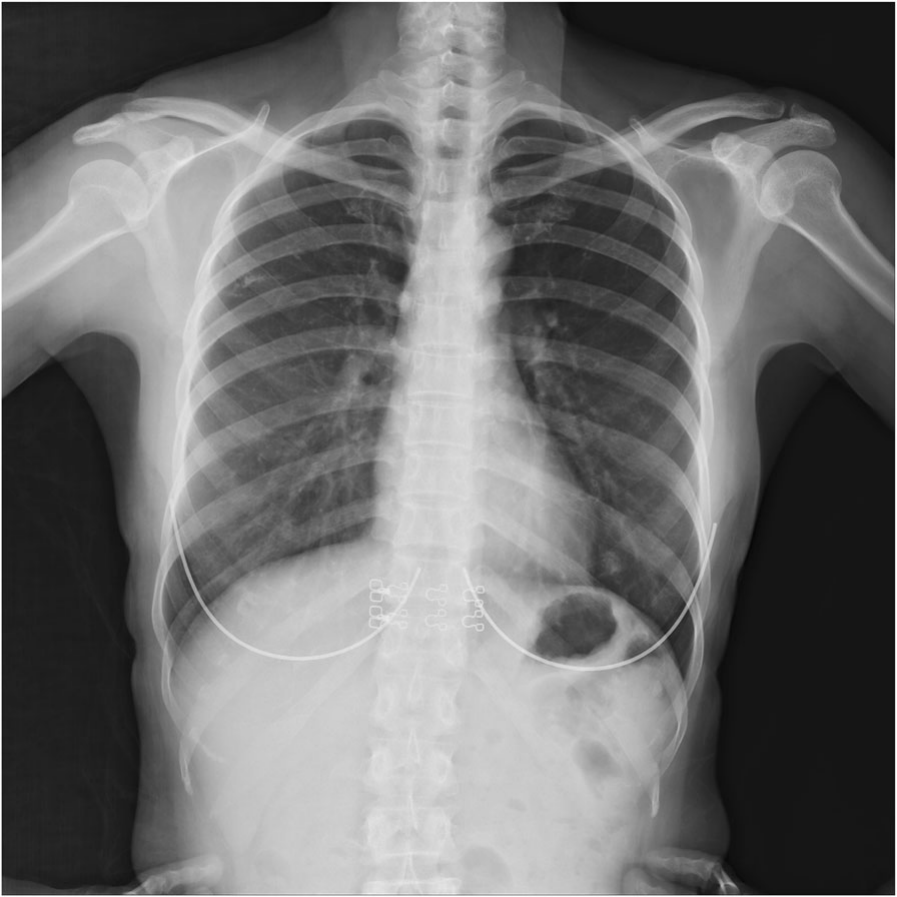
Initial chest radiograph. The initial chest radiograph shows a calcified nodule in the upper right pulmonary lobe (yellow arrowhead)
